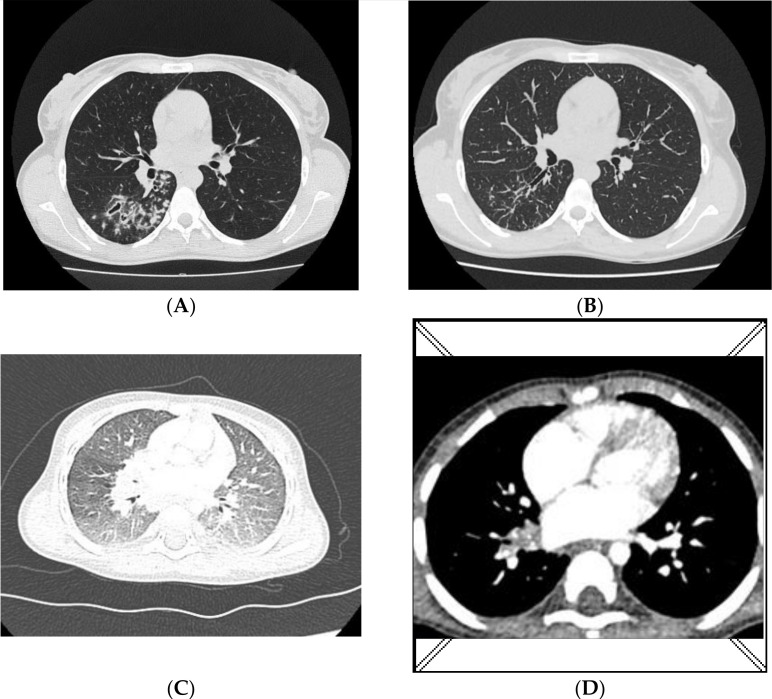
Chest CT A/B—mother; (A)—nodular lesions in the right lung (3 June 2020); (B)—regression of lesions (5 November 2020); C/D—infant; (C)—ground-glass opacities with some consolidations localized in both lungs (12 June 2020); (D)—regression of lesions, numerous calcifications in the nodes of the right hilum and subcarinal nodes (5 October 2020).
